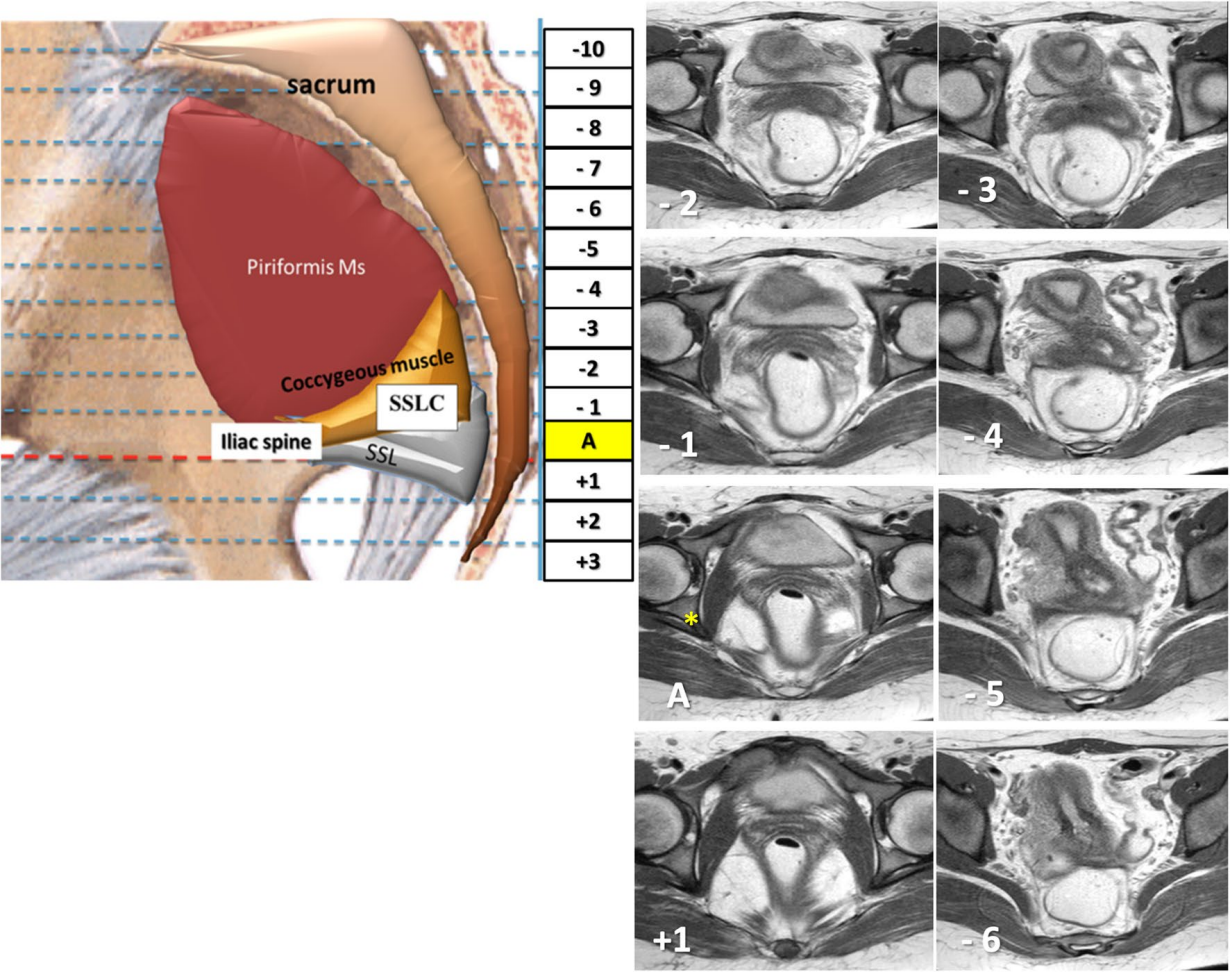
Grid system for uterosacral ligament evaluation: sequential MRI axial proton density-weighted images of a 25-year-old woman with urge urinary incontinence; image “A” was chosen to be the reference level and shows the tip of the ischial spine (*asterisk*); sequential images cephalad to image “A” were denoted with negative numbers and those caudad with positive numbers. *SSL* sacrospinous ligament, *SSLC* sacrospinous ligament-coccygeus muscle complex
